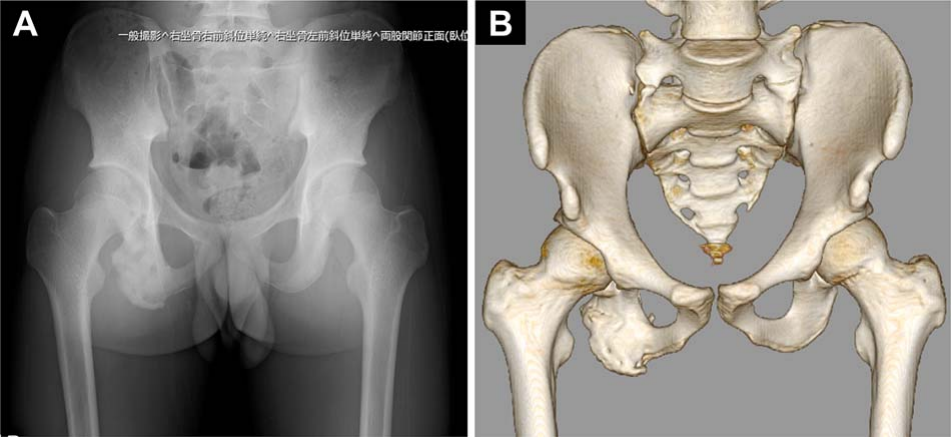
Postoperative images of the pelvis. (A) Plain radiograph. (B) Computed tomography scan.
